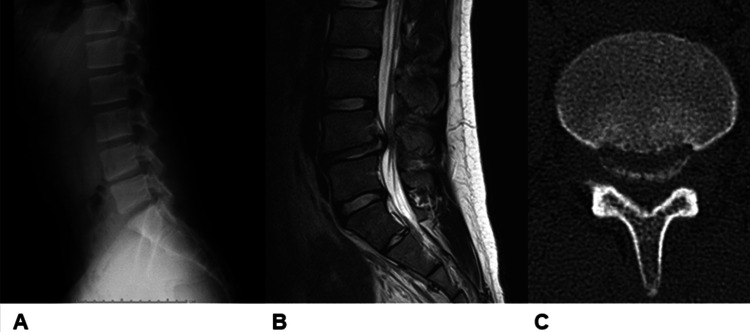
A case in which PRAF was difficult to diagnose by preoperative X-ray and MRI but could be diagnosed by a postoperative CT scan (case 20) A: A preoperative X-ray showed no obvious bone fragments; B: A preoperative MRI showed no obvious bone fragments; C: A postoperative computed tomography led to the diagnosis of Takata type I posterior ring apophyseal fracture
